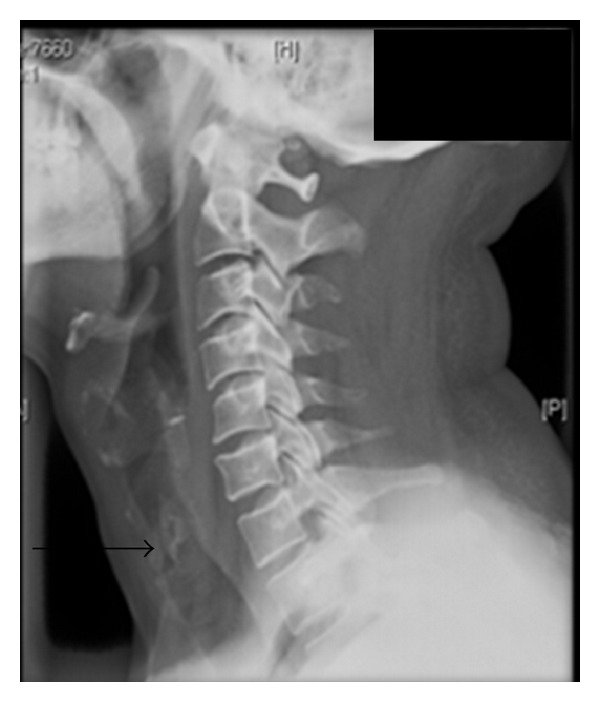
X-ray neck lateral view—radiopaque foreign body at C6-C7 level.
